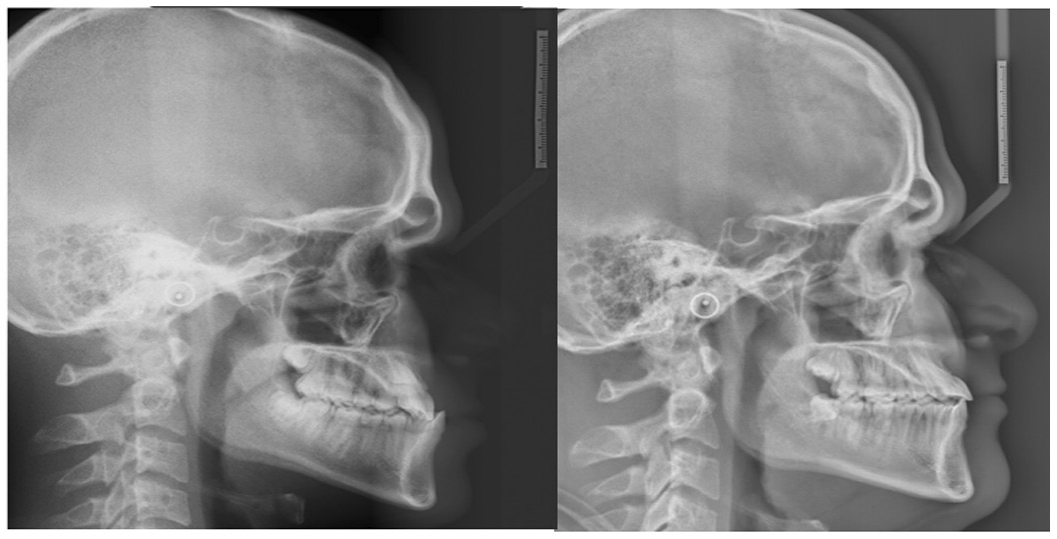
Pre and post‐treatment cephalometric radiographs.
